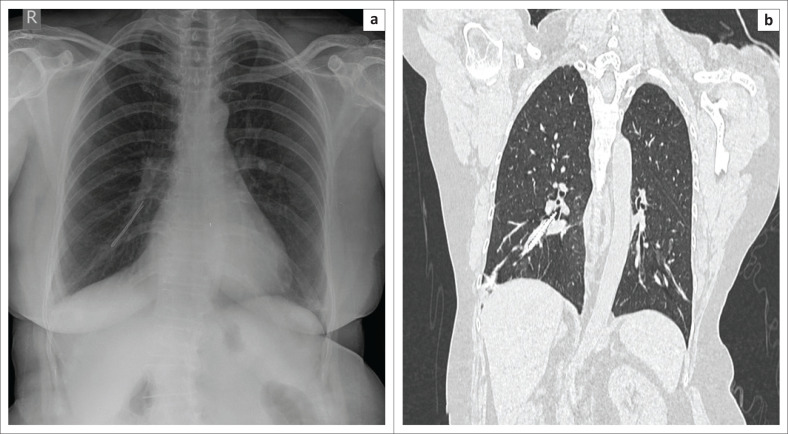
(a) Chest radiograph and (b) coronal reformatted chest computed tomography images of a 59-year-old lady who accidentally aspirated a hair pin (arrows) into the right lower lobe bronchus.
